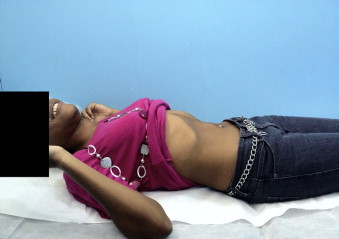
Index patient immediately post CT scanning demonstrating a flat abdomen.
